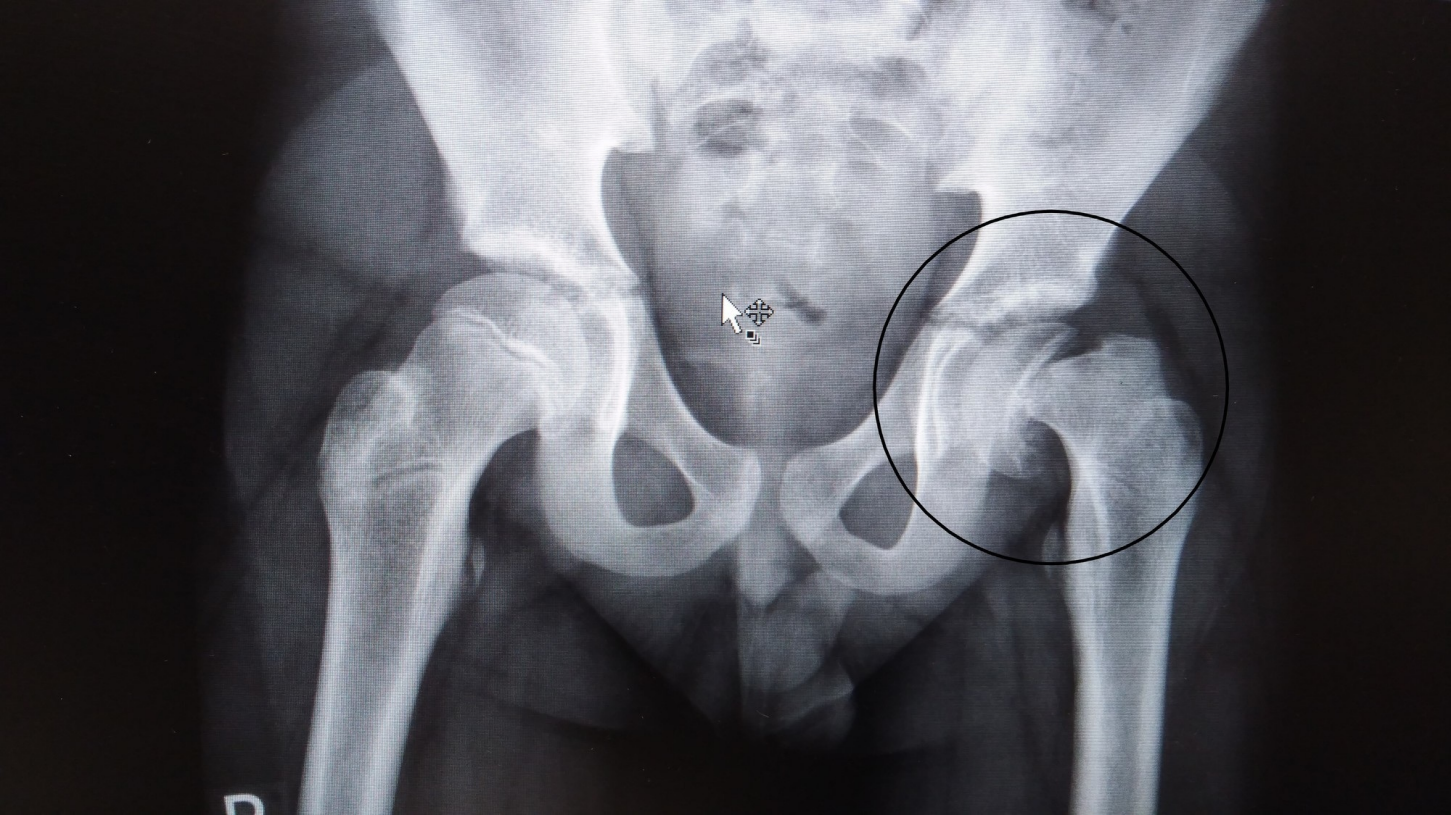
X-ray left hip with slipped epiphysis. X-ray showing complete slippage of the epiphysis.
